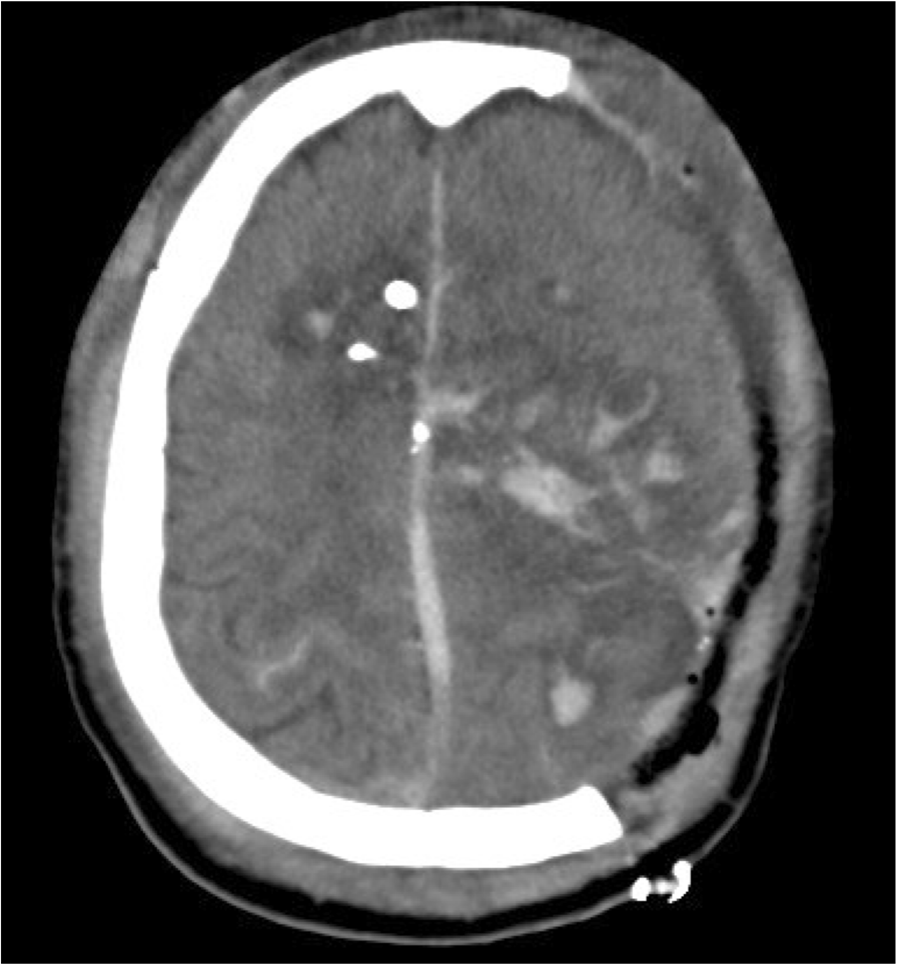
Computed tomography (CT) scan of patient with transhemispheric gunshot wound and associated intracranial hemorrhage
